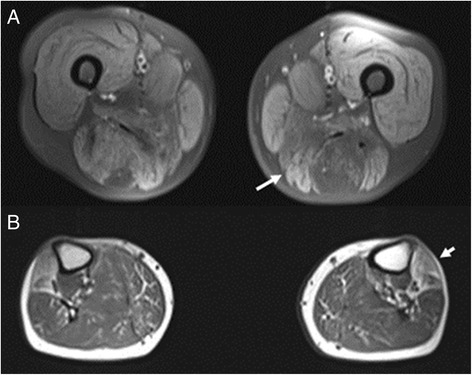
Axial MRI of the thigh (a) and the calf (b). a. T1 fatsat image shows fatty degeneration of muscles in the posterior compartment (dark, arrow). b. T1 image shows degeneration of anterior tibial muscles (arrow) and fatty infiltration of medial gastrocnemius muscles
